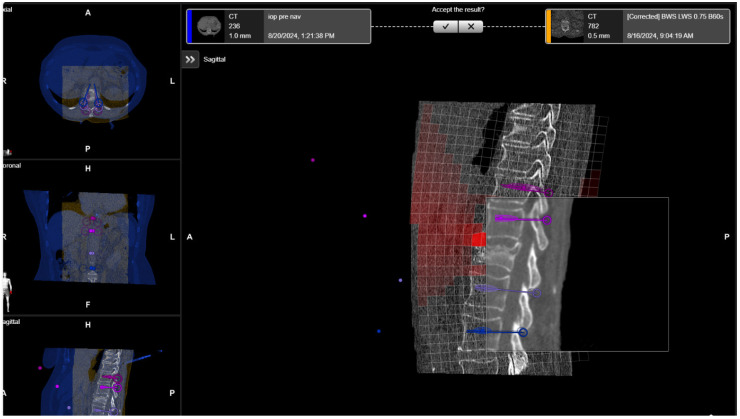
Elastic fusion of the preoperative CT and the iCT scan with preplanned screw trajectories in a fracture at the thoracic–lumbar junction, performed due to instability.
